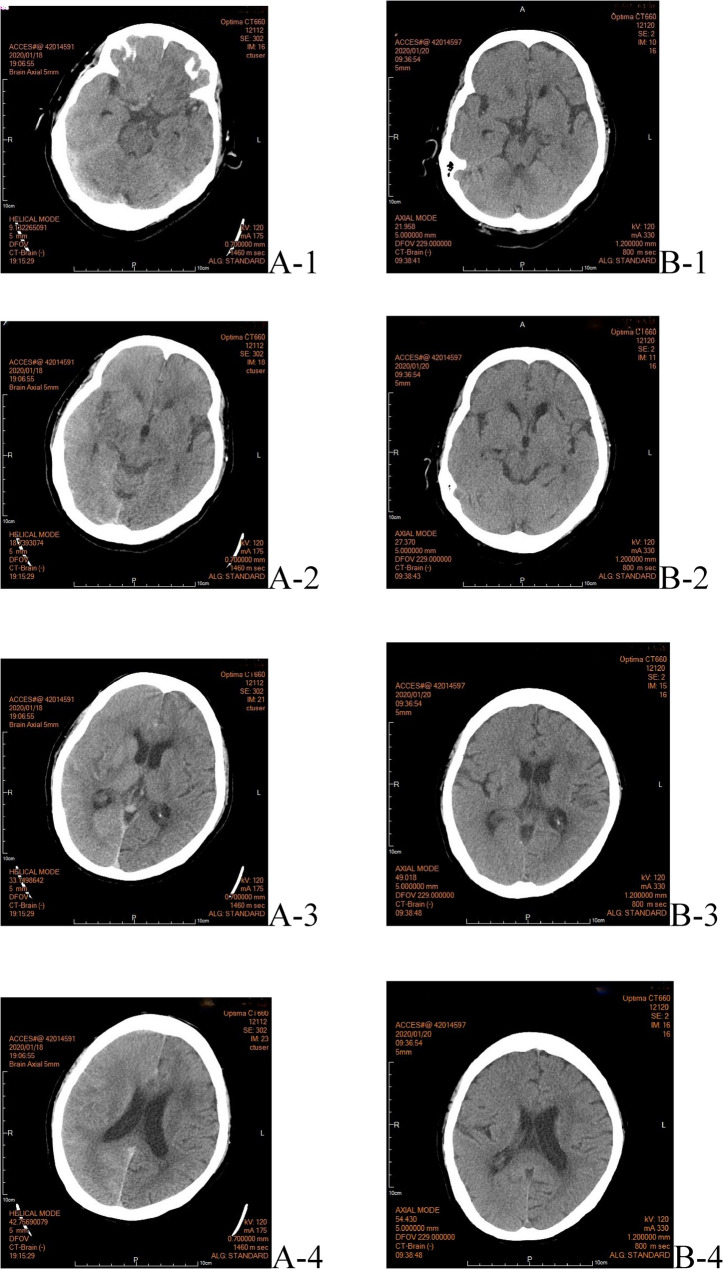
Serial non-contrast CT brain scan. A Immediate post-procedure CT reveals a hyperdense right MCA sign (arrow in A-1) and diffuse right hemispheric ‘white-out’ with effacement of the sulci (A-2, A-3,A-4). B Follow-up CT at 48 h demonstrated complete resolution of the hyperdensity and edema, with reappearance of normal sulcal patterns (B-2, B-3,B4). A-1 (CIE-1) at the pons level: right side dense MCA sign (+), diminished right side sulcus, and blurred gray-white matter junction with enhanced brightness over the right hemisphere. A-2 (CIE-2) at 3rd ventricle level/basal cisterna: diminished right-side sulcus and blurred gray-white matter junction with enhanced brightness over the right hemisphere. A-3 (CIE-3) at the lateral ventricle with anterior and posterior horns: disappearance of the right-sided sulcus and a blurred gray-white matter junction with enhanced brightness over the right hemisphere. A-4 (CIE-4) at the falx/genu and splenium of the Corpus Callosum level: showing disappearance of the right-side sulcus and blurred grey-white matter junction with enhanced brightness over the right hemisphere, as well as some linear hyperdensity over earlier sulci. Panel B Follow-up CT (48 h later) showing resolved edema.B-1 (PCIE-1) at the pons level as A-1: reappearance of some sulci with a still blurred gray-white matter junction. B-2 (PCIE-2) at 3rd ventricle level/basal cisterna as A-2: re-appearance of some Sulci, with more prominent Sylvian fissure and disappearance of right hemi-sphere “white-out” B-3 (PCIE-3) at the lateral ventricle with anterior and posterior horns as A-3: reappearance of some sulci of the frontal lobe with a still blurred gray-white matter junction. B-4 (PCIE-4) at Falx and genu and splenium of Corpus Callosum level: re-appearance of some Sulci over right hemisphere (still less than left side) (Generally speaking , right hemisphere diffuse “ white-out” is clearly appreciated over A-2, A-3, and A-4, and it disappeared in B-2, B-3, and B-4.)
